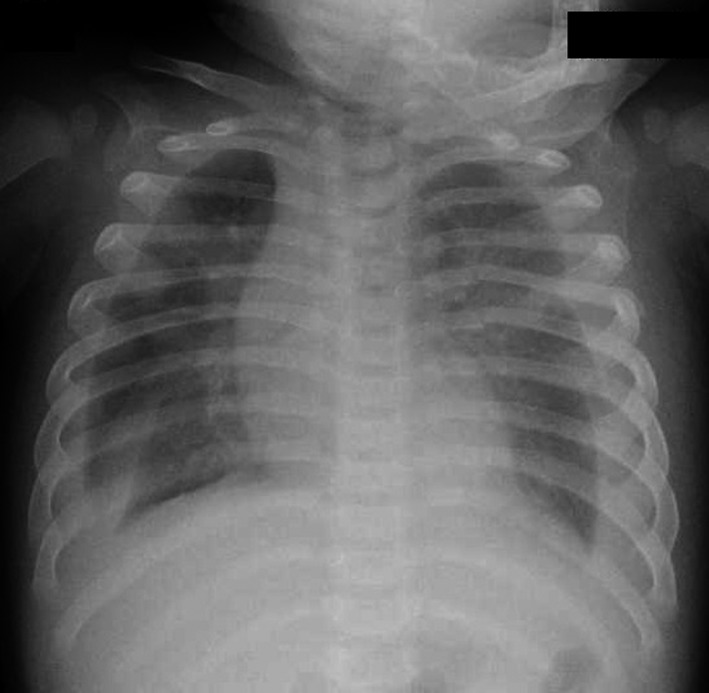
Chest radiography shows bilateral pleural fluid.
